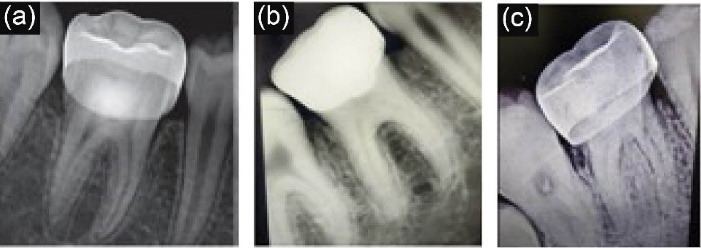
Table 2 T2: Radiographic evaluation at follow-up time interval of 6 and 12 and 24 months
